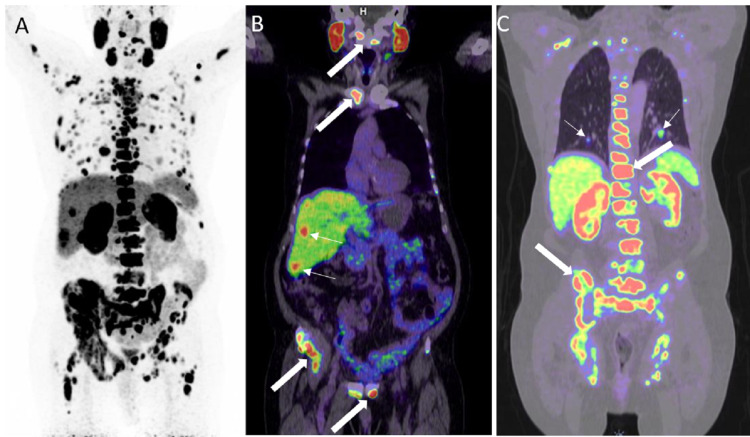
Extensive bone, liver, and lung metastases A: 68Ga PSMA PET MIP image shows extensive metastases in the bones, liver, and lungs. B: Coronal PET/CT in the soft tissue window in the same patient shows liver metastases (white thin arrows). Also, note the multiple bone metastases (white thick arrows). C: Coronal PET/CT in the lung window in the same patient shows bilateral lung metastases (white thin arrows). Also, note the extensive bone metastases (white thick arrows).
